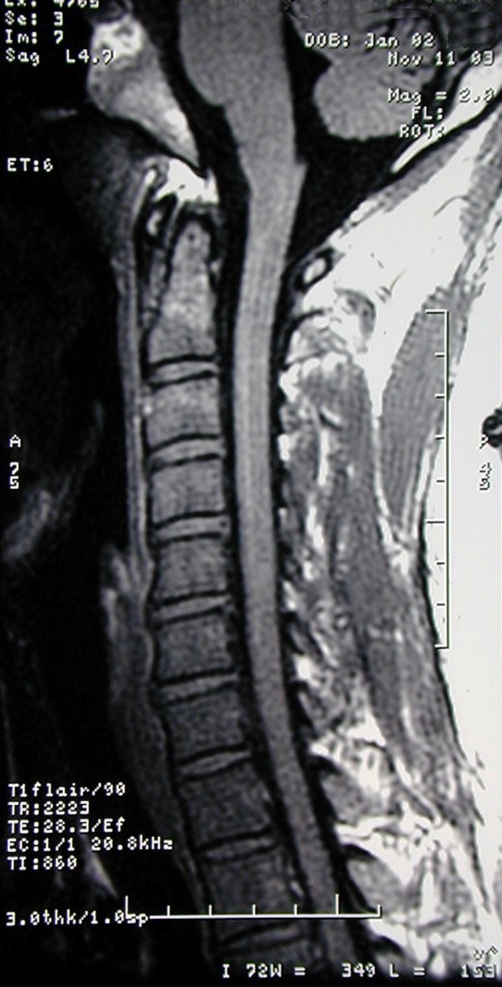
Normal cervical spine T1 weighted MRI.
